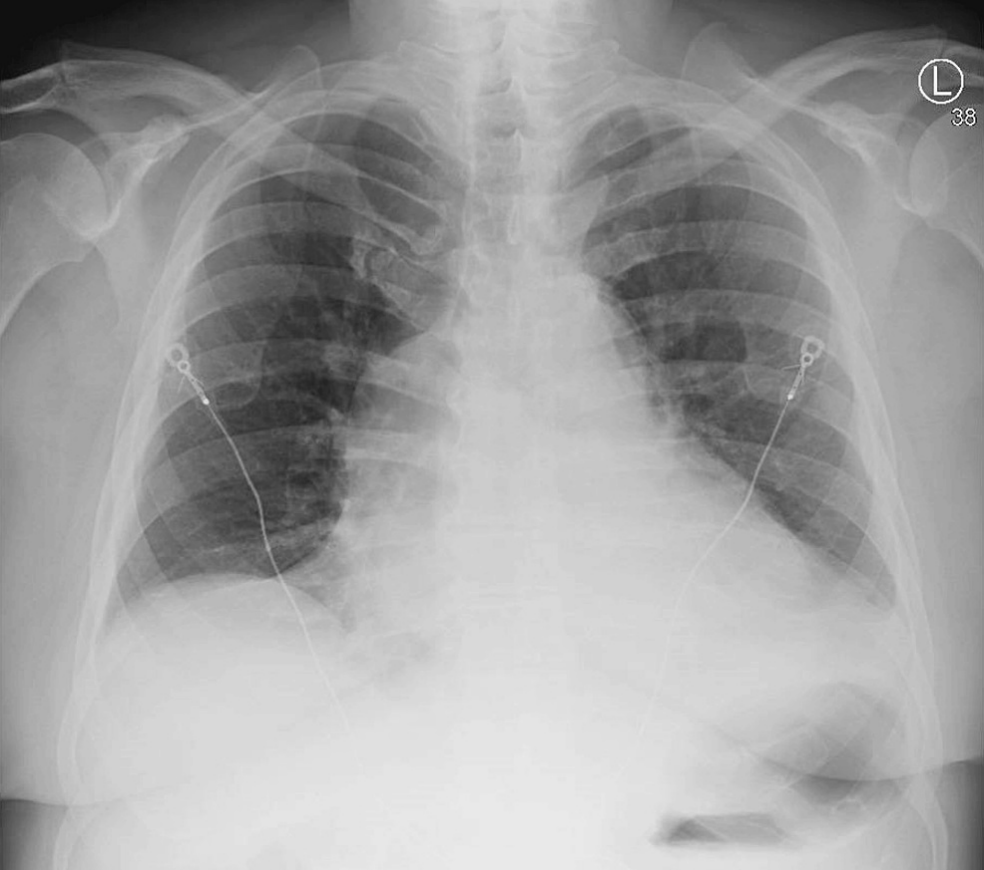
Chest X-ray (PA view) PA: Posterianterior
